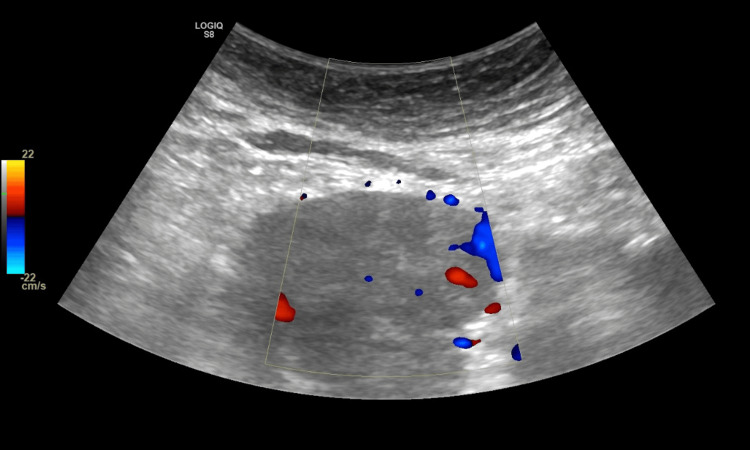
Abdominal ultrasound showing mass. Several mesenteric vessels can be identified anterior to the lower abdominal mass.
